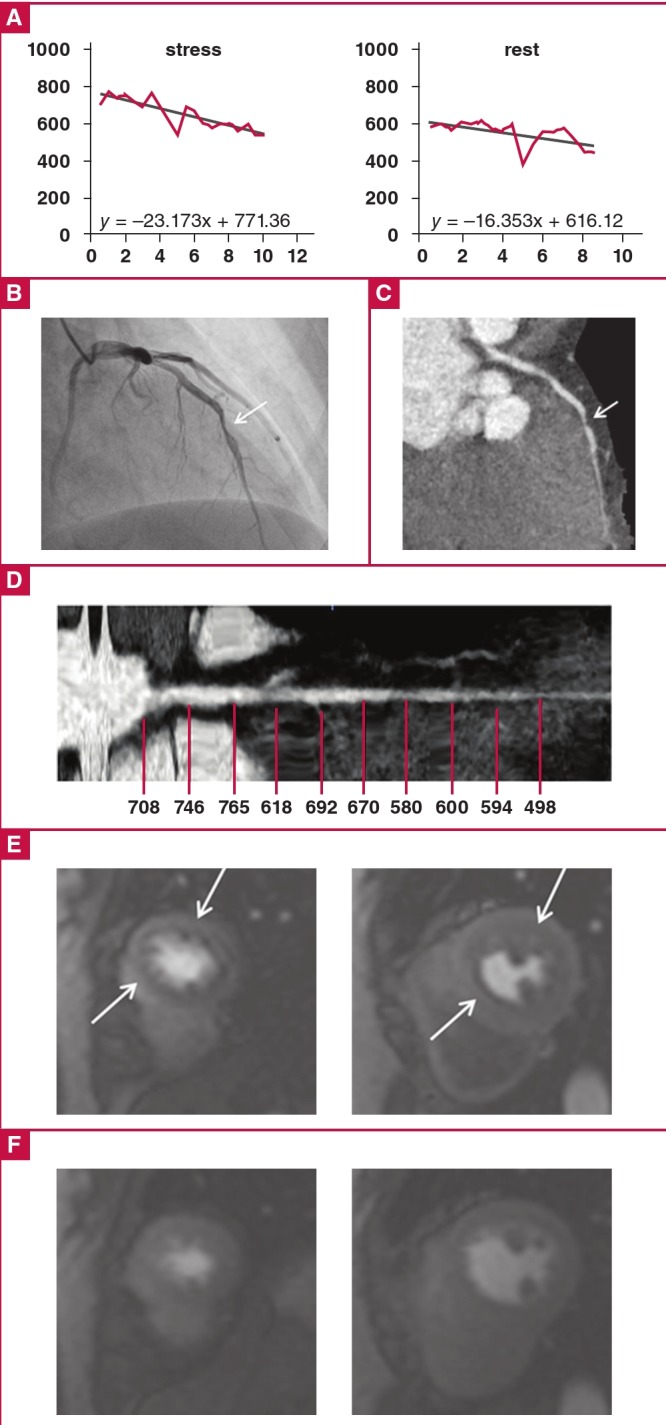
A 47-year-old woman with chest pain. (A) TAG of the LAD was –23 HU/10 mm on stress CCTA and –16 HU/10 mm on rest CCTA. (B) Invasive coronary angiography, and (C) curved multi-planar reformatted images of stress CCTA show significant stenosis in the mid-LAD. (D) Axial multi-planar reformatted image shows corresponding luminal attenuation of CCTA. (E) CMR imaging with stress perfusion imaging shows low signal intensity, indicating a subendocardial perfusion defect in the anterior septum and anterior wall at the mid-ventricular level (LAD territory). (F) No low-signal intensity lesion was visible with rest perfusion imaging. TAG = transluminal attenuation gradient, LAD = left anterior descending artery, CCTA = coronary computed tomography angiography, CMR = cardiac magnetic resonance.
